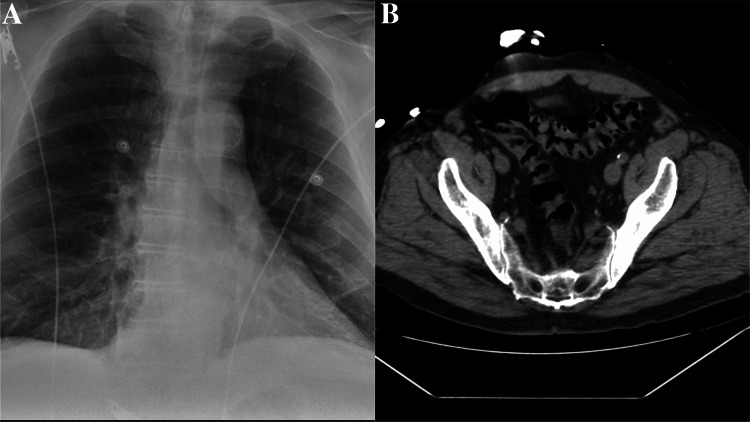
(A) Emphysematous changes in the chest x-ray and (B) diverticulosis revealed in abdominal CT.
